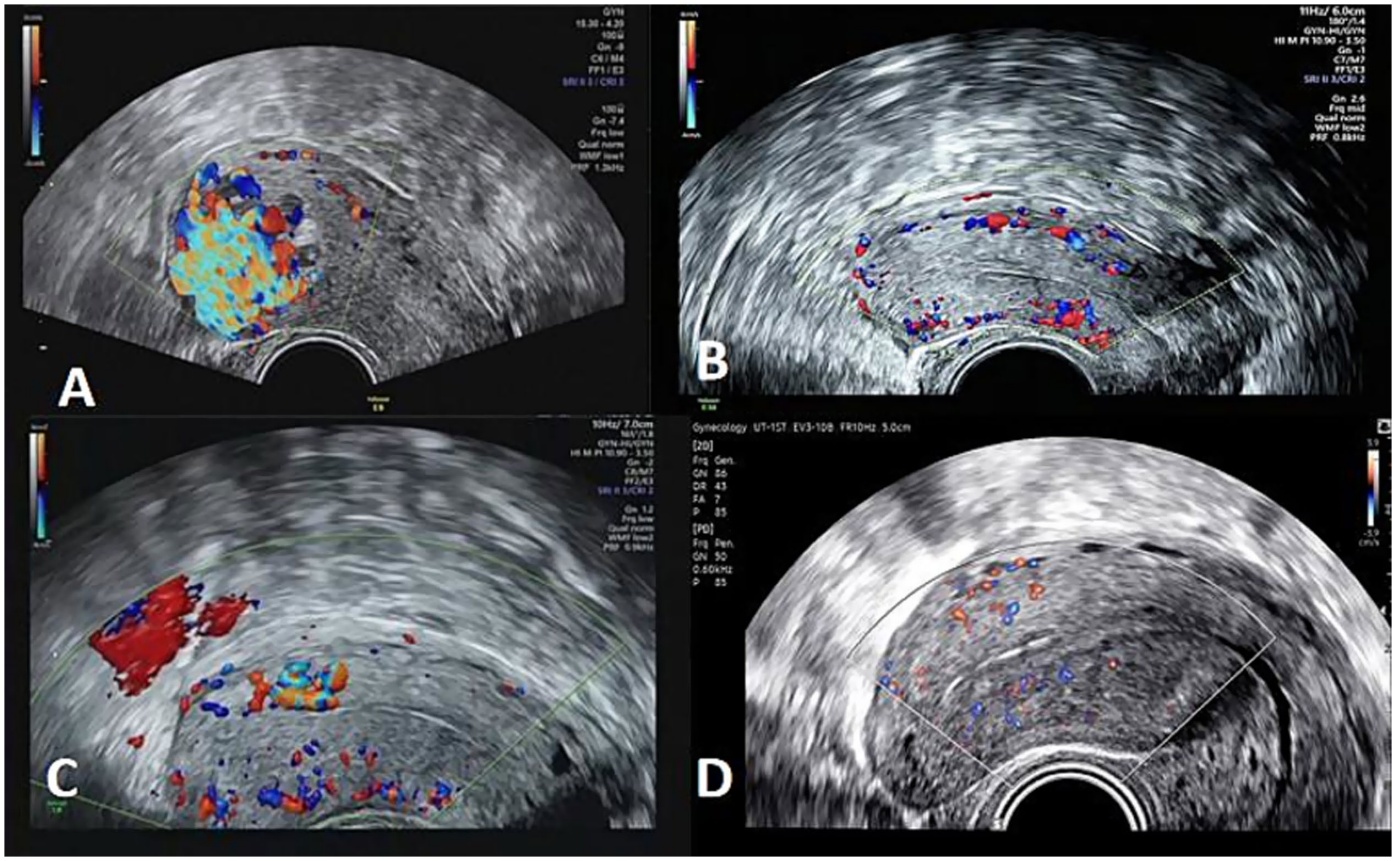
Ultrasound images of two patients before and after drug treatment. (A,C) Color Doppler ultrasound images of blood flow before treatment for patients in Patients 1 and 2, respectively. (B,D) Blood flow ultrasound images after treatment.
